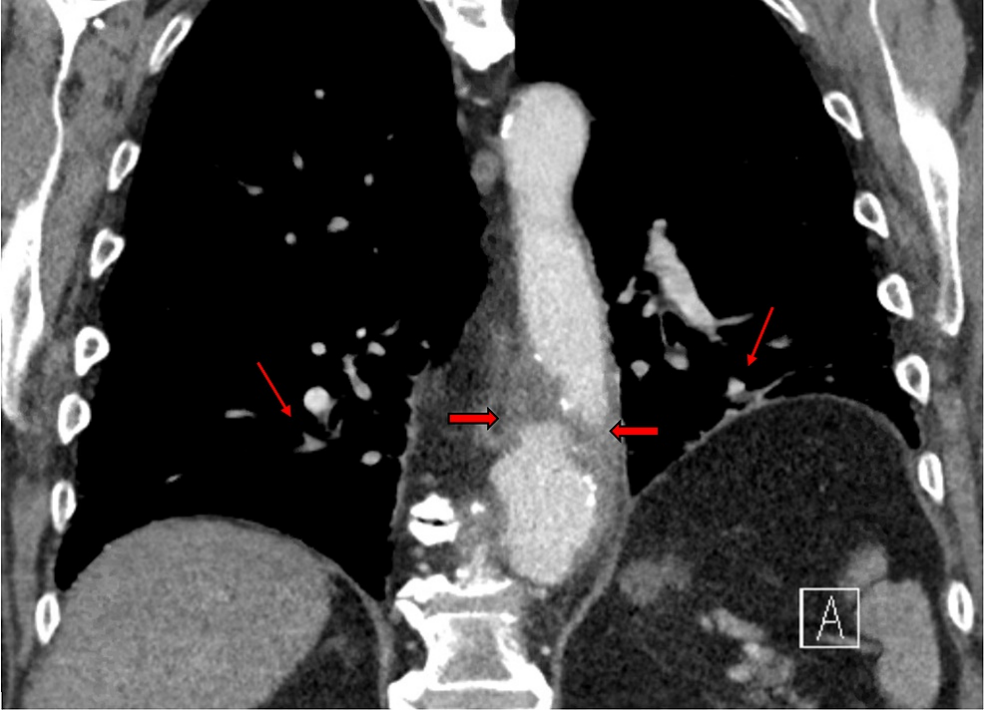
CT of the chest with contrast revealed improving multilobar pneumonia (oblique red arrows) and a new aneurysmal dilatation of the thoracic aorta with an acute dissection (horizontal red arrows). The aorta size measured 3.9 x 4.4 cm in the transverse dimension compared to 2.9 x 3 cm previously. The aorta margins were partially ill-defined and clearly at risk for rupture.
